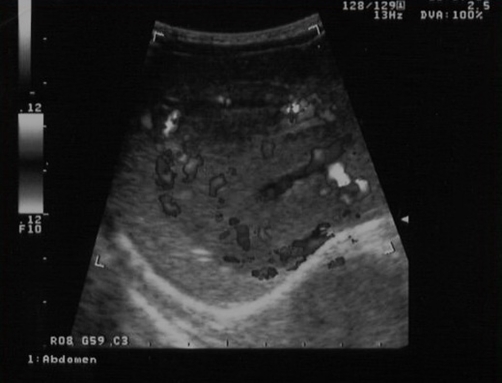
Hepatic lesion at postnatal ultrasound; marked, peripheral Doppler blood flow.
